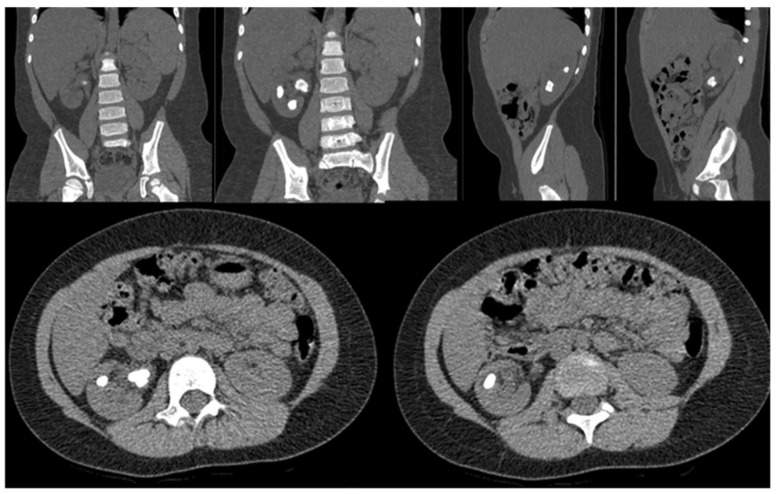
Abdominal CT scan showing right nephrolithiasis in a kidney affected by right UPJO.
